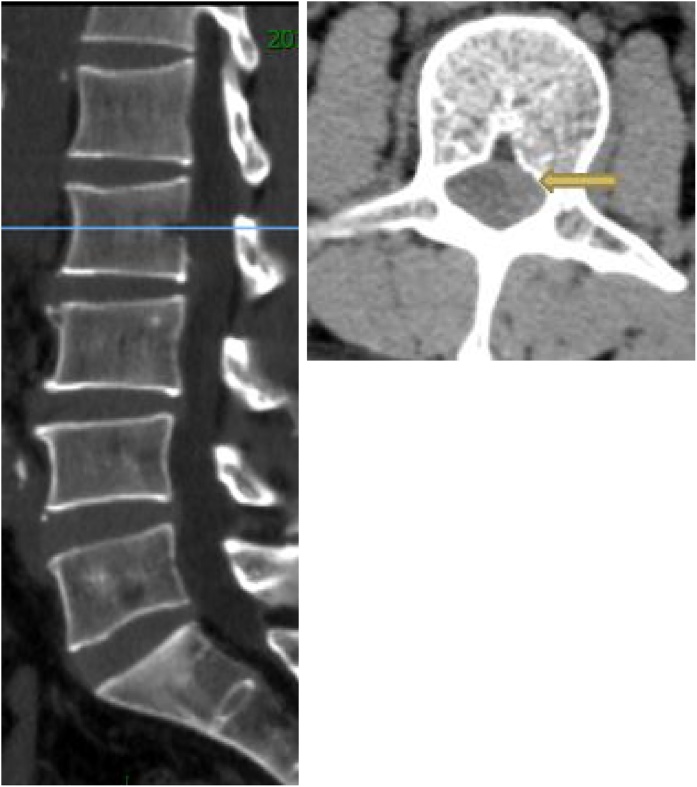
CT scans showed no intervertebral disc calcification was observed.
